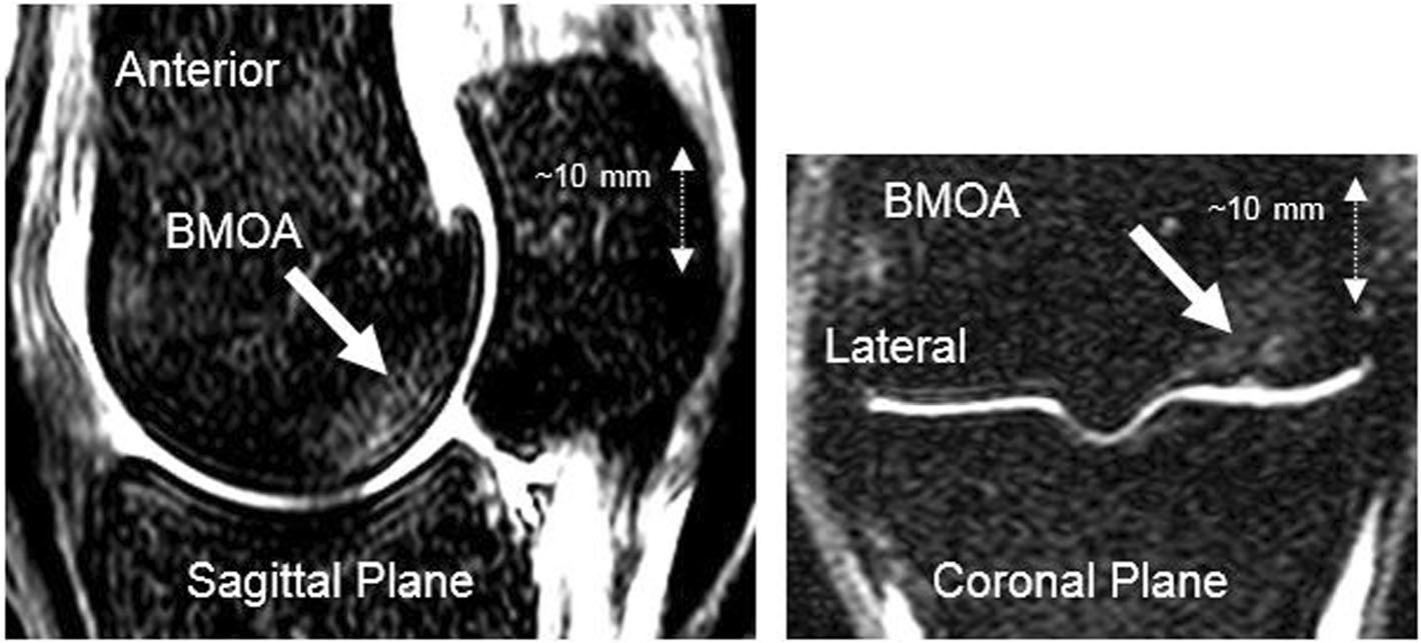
Coronal and midline sagittal magnetic resonance images of the metacapophalangeal joint. Arrows indicate the region of the BMOA
